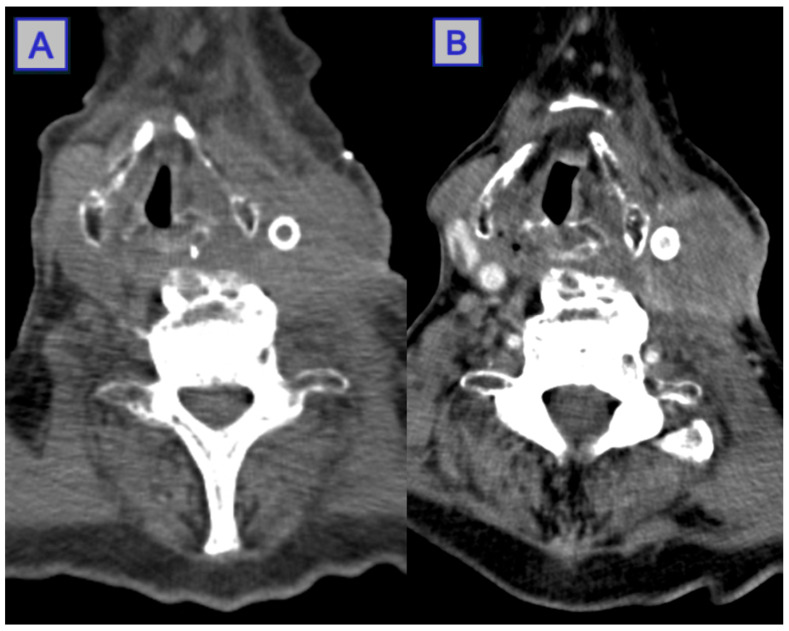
Images of CT scans showing a peri-carotid and left vocal cord lesion causing a shift of the midline. Pre-PDT (A), contrast enhanced scan with stent insertion. Post-PDT (B), a reduction of midline shift is noted, with evidence of necrotic and inflammatory tissue surrounding the tumour. Reprinted with permission from ref. [81]. Copyright 2010 Photodiagnosis Photodyn. Ther.
